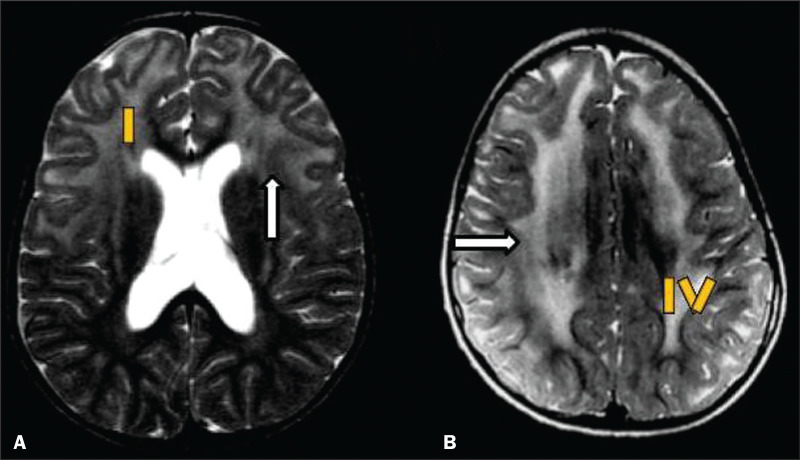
White matter involvement in INMDs. Axial MRI of the skull, correlated
